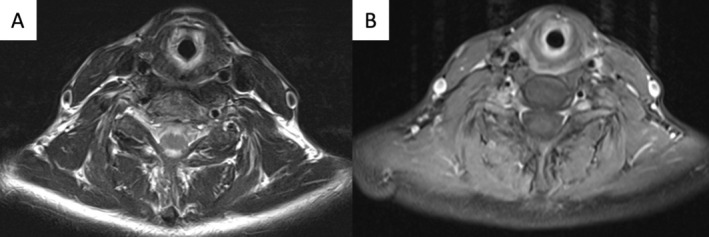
Magnetic resonance imaging of the subglottic region: T2‐weighted image (Panel A) shows a subglottic circumferential stenosis with mucosal thickening and T1‐weighted image (Panel B) demonstrates diffuse contrast enhancement.
